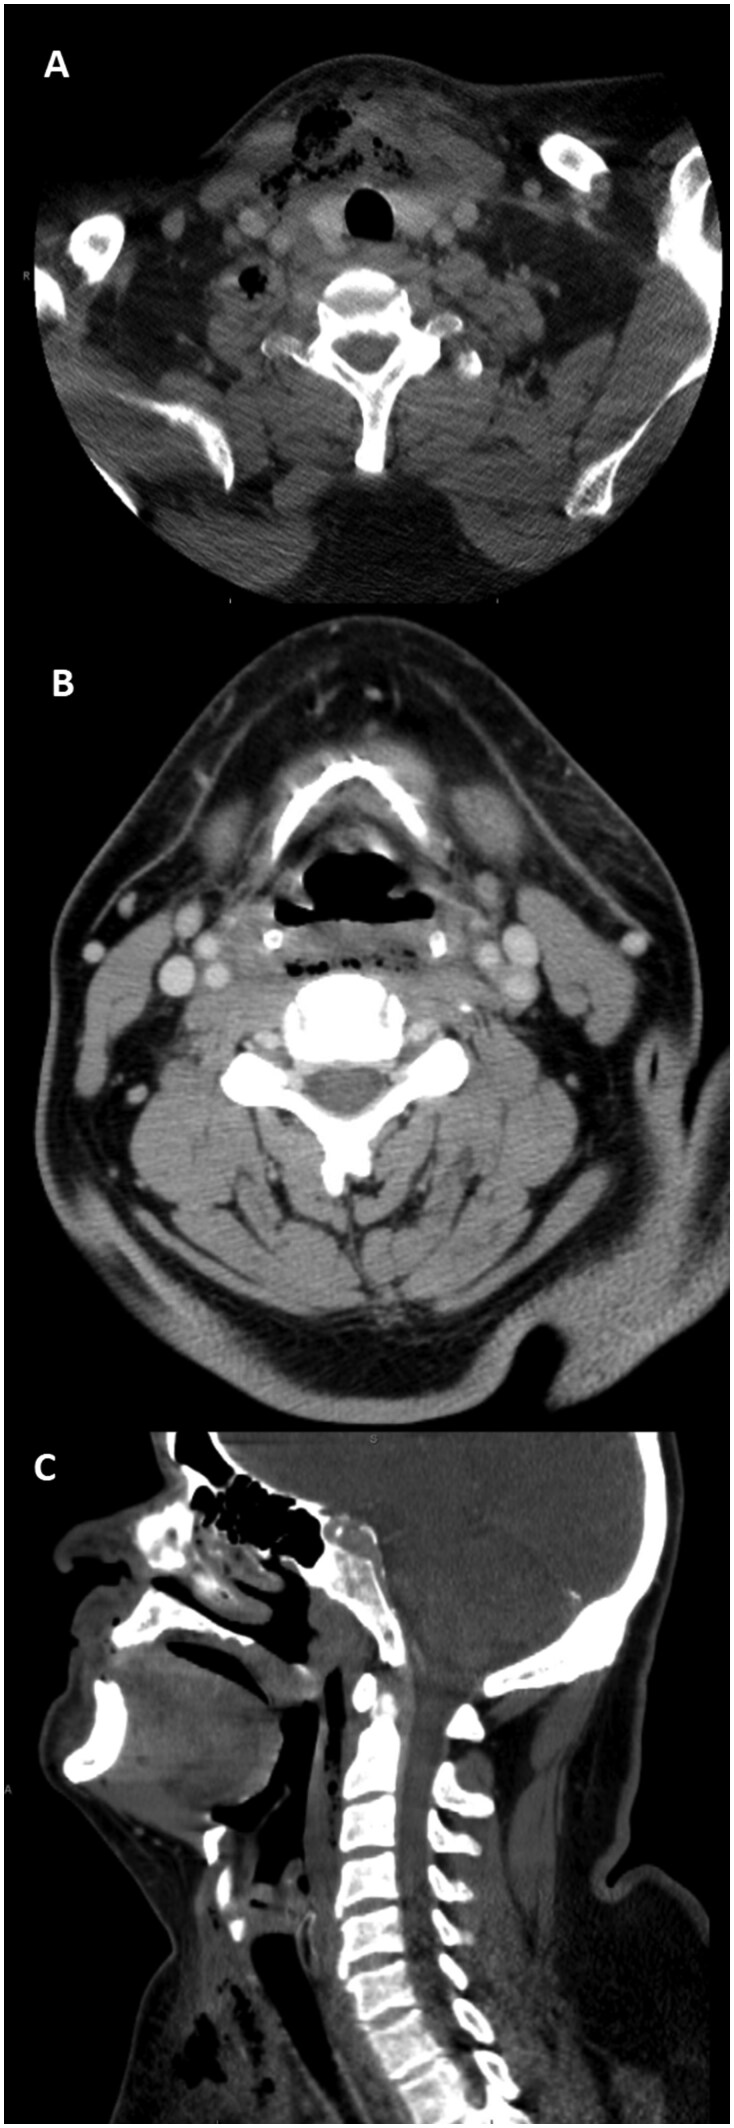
Contrast-enhanced axial (A and B) and coronal (C) CT of the neck demonstrate emphysema of the deep soft tissue of the anterior lower neck involving the strap muscles and extending to the right supraclavicular and infraclavicular region with fat stranding and retropharyngeal emphysematous effusion. Highly concerning for extensive, necrotizing fasciitis.
